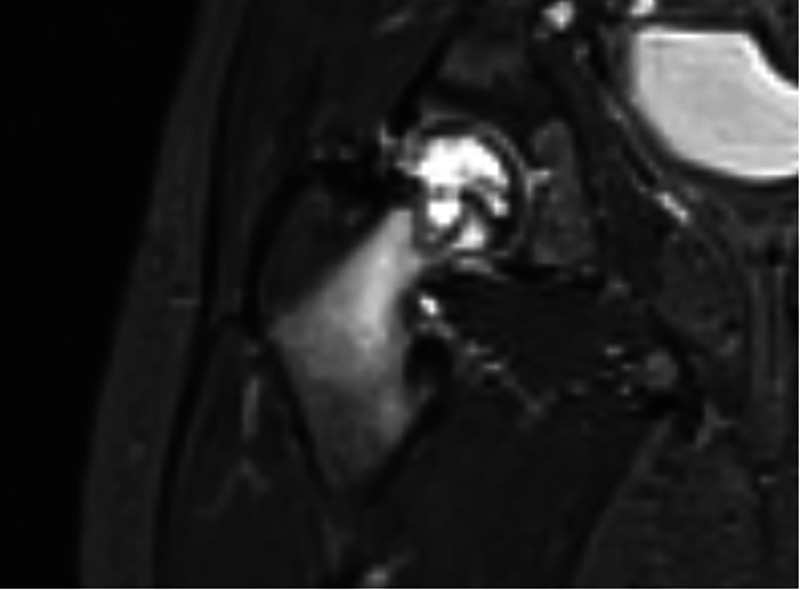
A 3-year-old child was referred to our emergency department with a limp for more than 3 weeks, without fever at admission, and the conventional radiography did not show any lesions. The laboratory tests were normal except for a sedimentation rate of 14 mm/h. MRI revealed a transphyseal lytic lesion of the femoral head without breach of the articular cartilage. A biopsy of the lesion under fluoroscopy detected the presence of *Kingella kingae* DNA.
